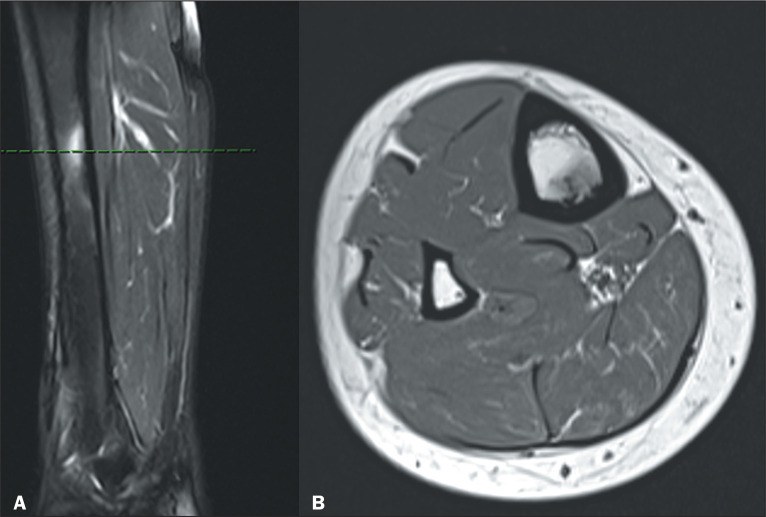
Magnetic resonance imaging of the lower limb of the patient in Figure 4, sagittal T2-weighted
